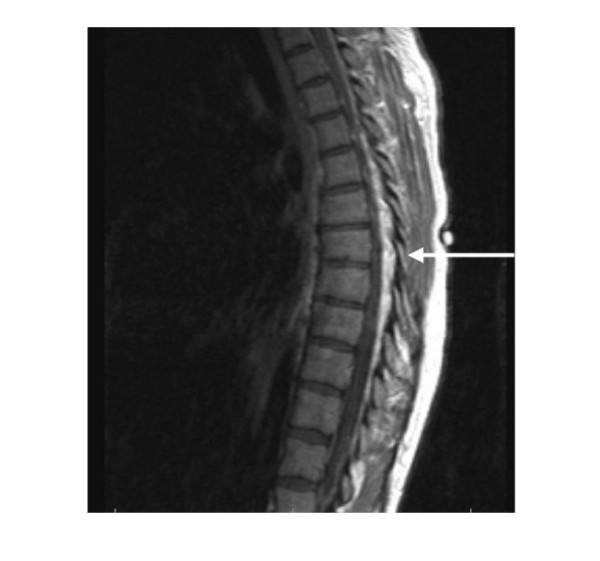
multi-planar, multi-sequence MRI imaging of the thoracic spine. Pre and post contrast sagittal and axial T1, and turbo T2 sequences acquired. An irregular masslike density predominantly T1 and T2 hyperintense with a serpiginous contour is demonstrated in epidural location, as a mass surrounding the thoracic cord. The lesion extends from the T1 level distally through the lower thoracic spine.
